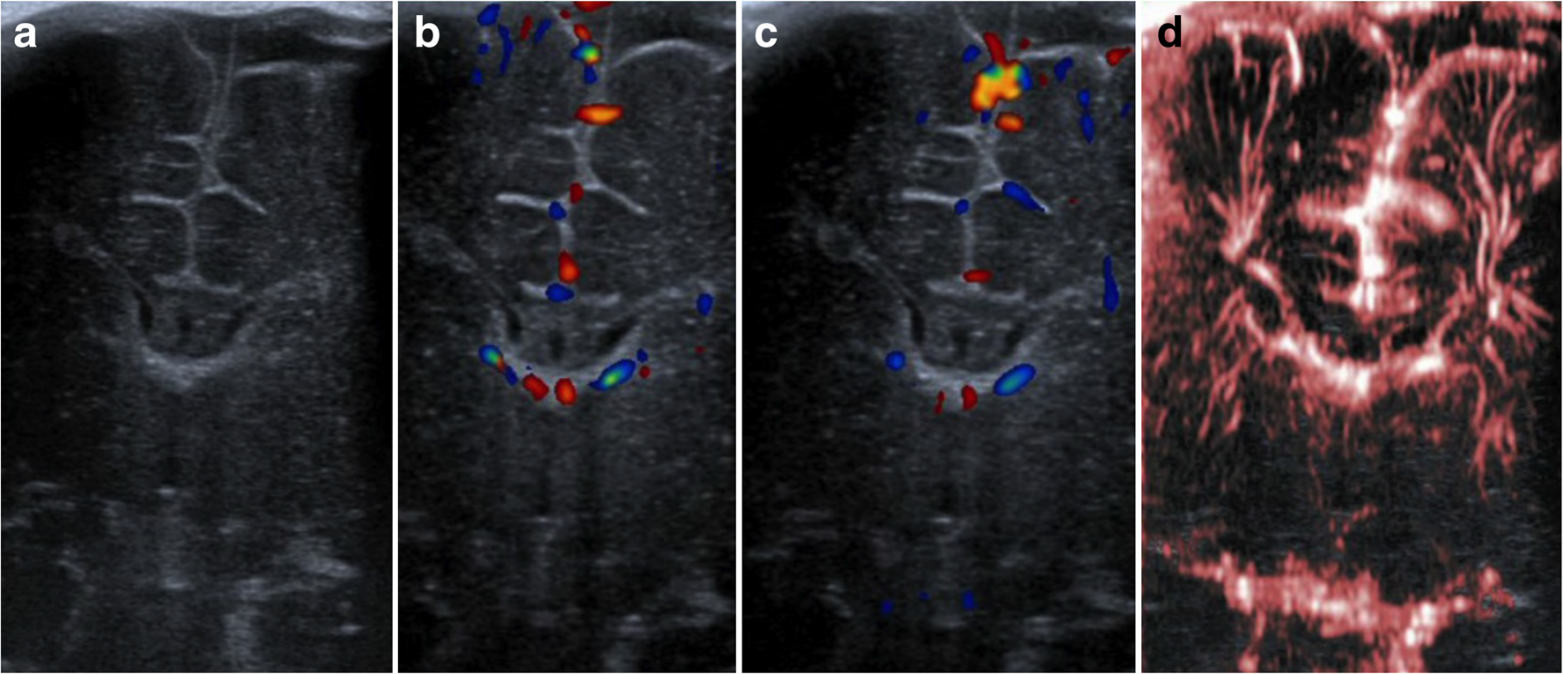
Comparison between clinical ultrasound and SMI: coronal deep view in B-mode (a), normal colour Doppler ultrasound (b, c), and colour SMI (d)
